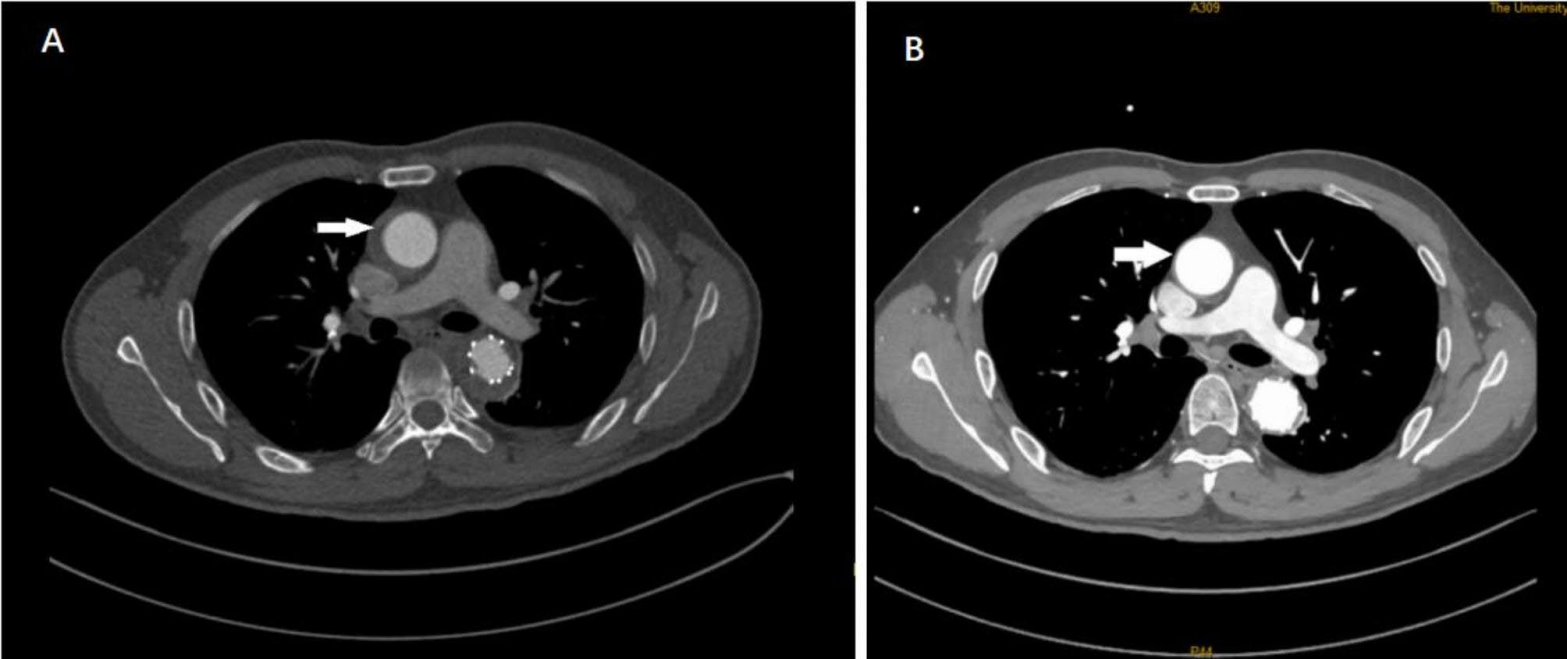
A Follow-up CT scan, 2 weeks postoperatively with resolution of type A IMH and satisfactory positioning of the TEVAR. Image showing that the ascending aortic hematoma is smaller than before. B Follow-up CT scan, 3 months postoperatively with resolution of the type A IMH. Image showing ascending aortic hematoma were completely absorbed
